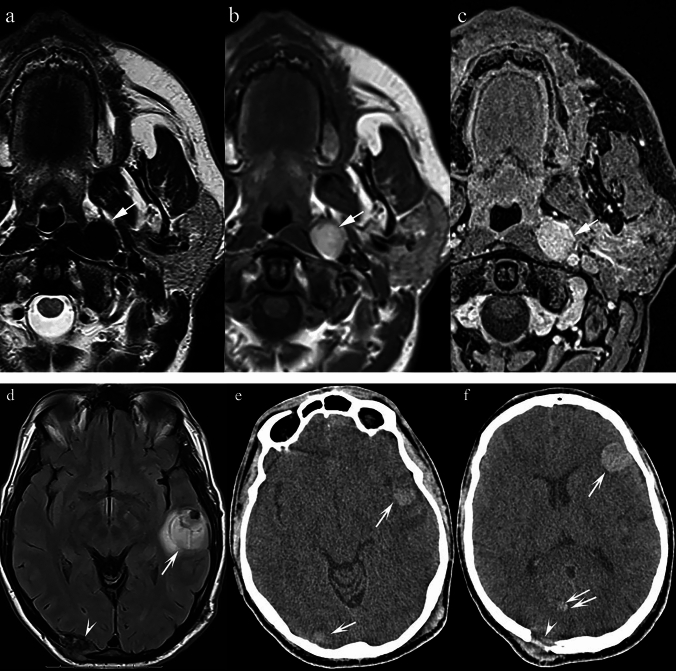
Patient with a history of lower lip melanoma for which he underwent local excision accompanied by radical bilateral neck dissection. There was no history of thyroid malignancy. a Axial T2 TSE at the level of the first cervical vertebra reveals a modestly sized, well-demarcated, low signal intensity mass in the left parapharyngeal space which is clearly separate from the tail of the parotid gland (arrow). b Axial T1 SE sequence without contrast at the same level reveals the lesion to be of very high signal intensity relative to the parotid gland (arrow). c T1 VIBE post-contrast demonstrates homogenous enhancement (arrow). d FLAIR sequence of the brain demonstrates a large haemorrhagic metastasis in the left temporal lobe (arrow) and a cortical breach in the occipital bone on the right side due to another metastatic deposit (arrowhead) e Non-contrast CT scan at the level of the midbrain, demonstrates a haemorrhagic metastasis in the left temporal lobe (single arrow) and a metastasis in the right occipital lobe (double arrow). f Non-contrast CT scan at the level of the thalamus, shows a haemorrhagic metastasis in the left frontal lobe (single arrow), in the right occipital lobe (double arrow) and a bone metastasis in the occipital bone on the right side (arrowhead)
